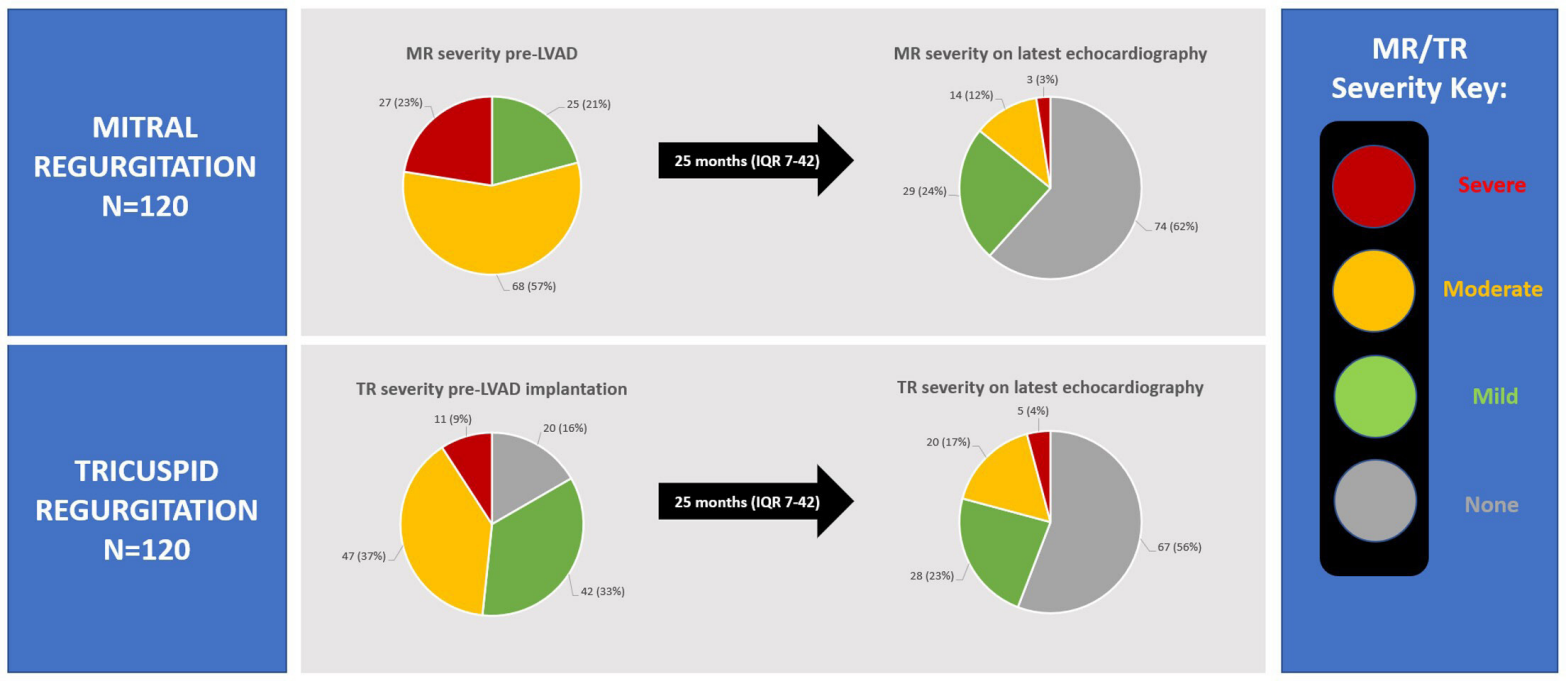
Pie charts demonstrating change in mitral and tricuspid regurgitation severity on echocardiography from pre-LVAD implantation to latest follow-up scan (n=120). LVAD, left ventricular assist device; MR, mitral regurgitation; TR, tricuspid regurgitation.
